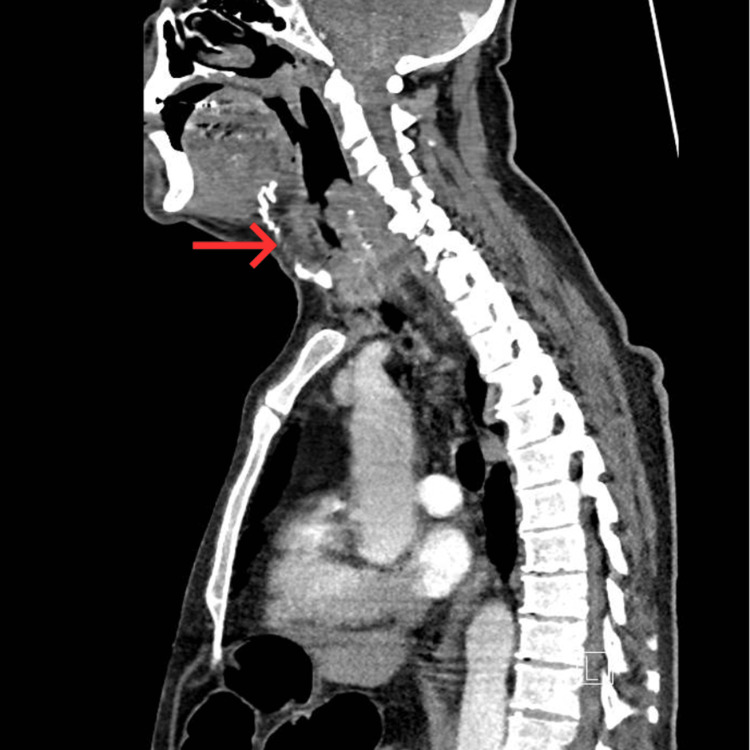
Sagittal CT of the neck demonstrating severe supraglottic and pharyngeal oedema. The red arrow highlights marked soft-tissue swelling with significant narrowing of the upper airway.
